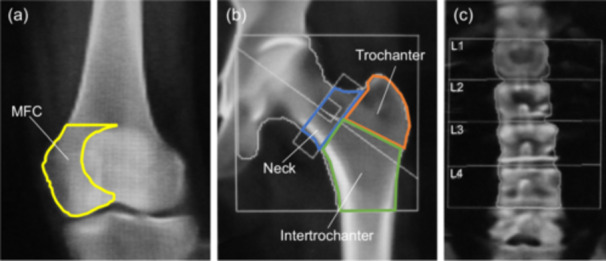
BMD measurement of each skeletal region using DEXA in female patients diagnosed with MMPRT on MRI. (a) BMD of the MFC (yellow line) was measured by defining a region from the superior border of the patella to the MFC, excluding the overlapping portion of the patella. (b) BMD of the proximal femur was measured at the femoral neck (blue line), trochanteric region (orange line), intertrochanteric region (green line) and the total hip region. (c) BMD of the lumbar spine was measured from L1 to L4. BMD, bone mineral density; DEXA, dual‐energy X‐ray absorptiometry; MFC, medial femoral condyle; MMPRT, medial meniscus posterior root tears; MRI, magnetic resonance imaging.
